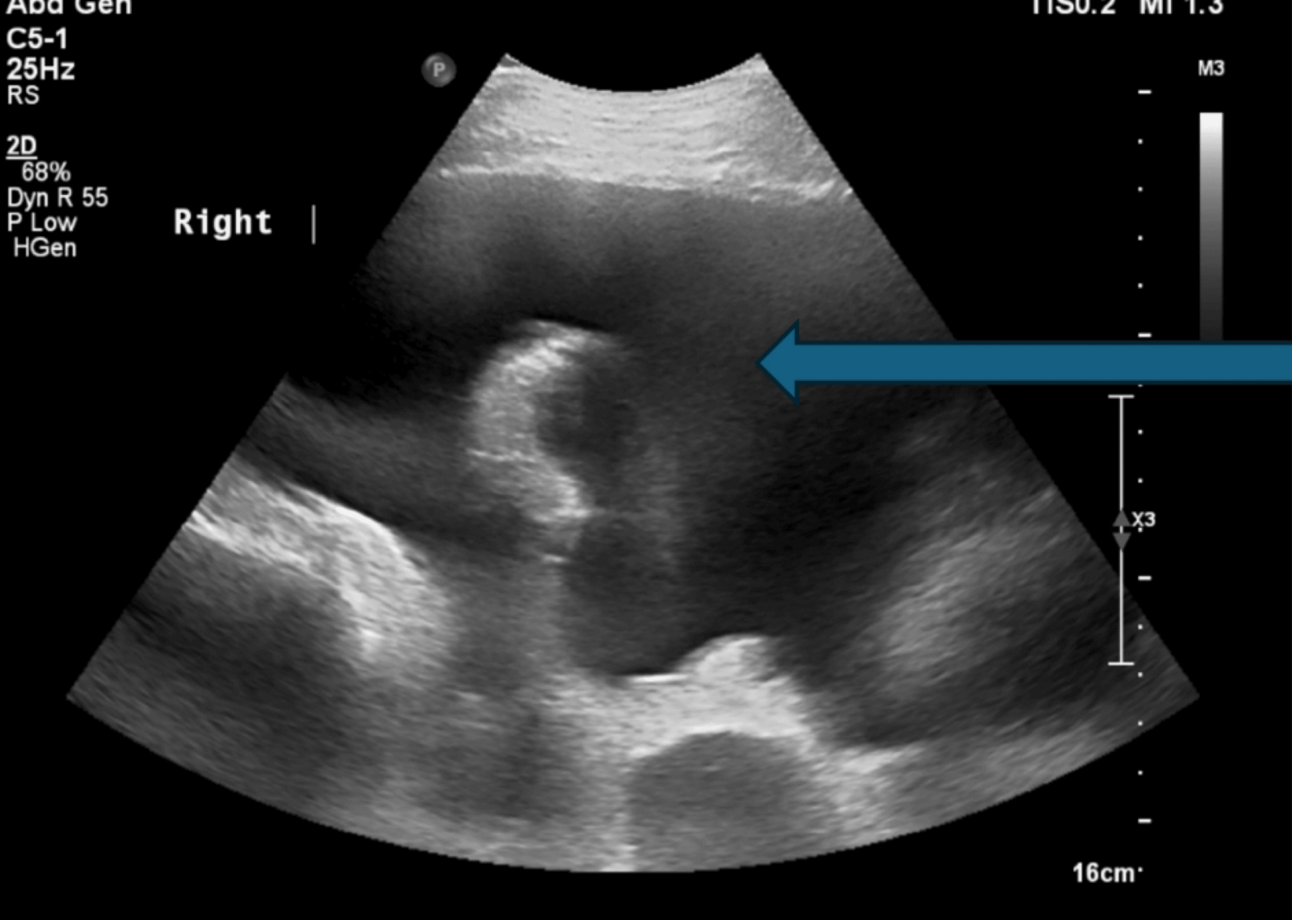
Right pleural effusion evident on ultrasound-guided thoracentesis in May 2025
